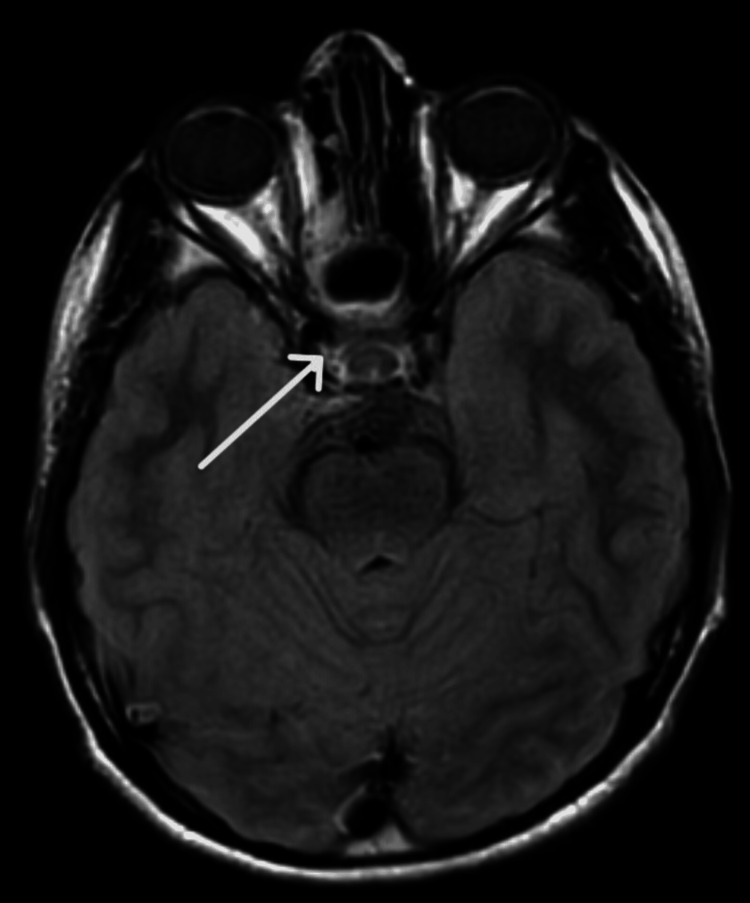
Postoperative second-month contrast-enhanced MRI scan of patient number 6 showing the complete excision of juvenile nasopharyngeal angiofibroma
